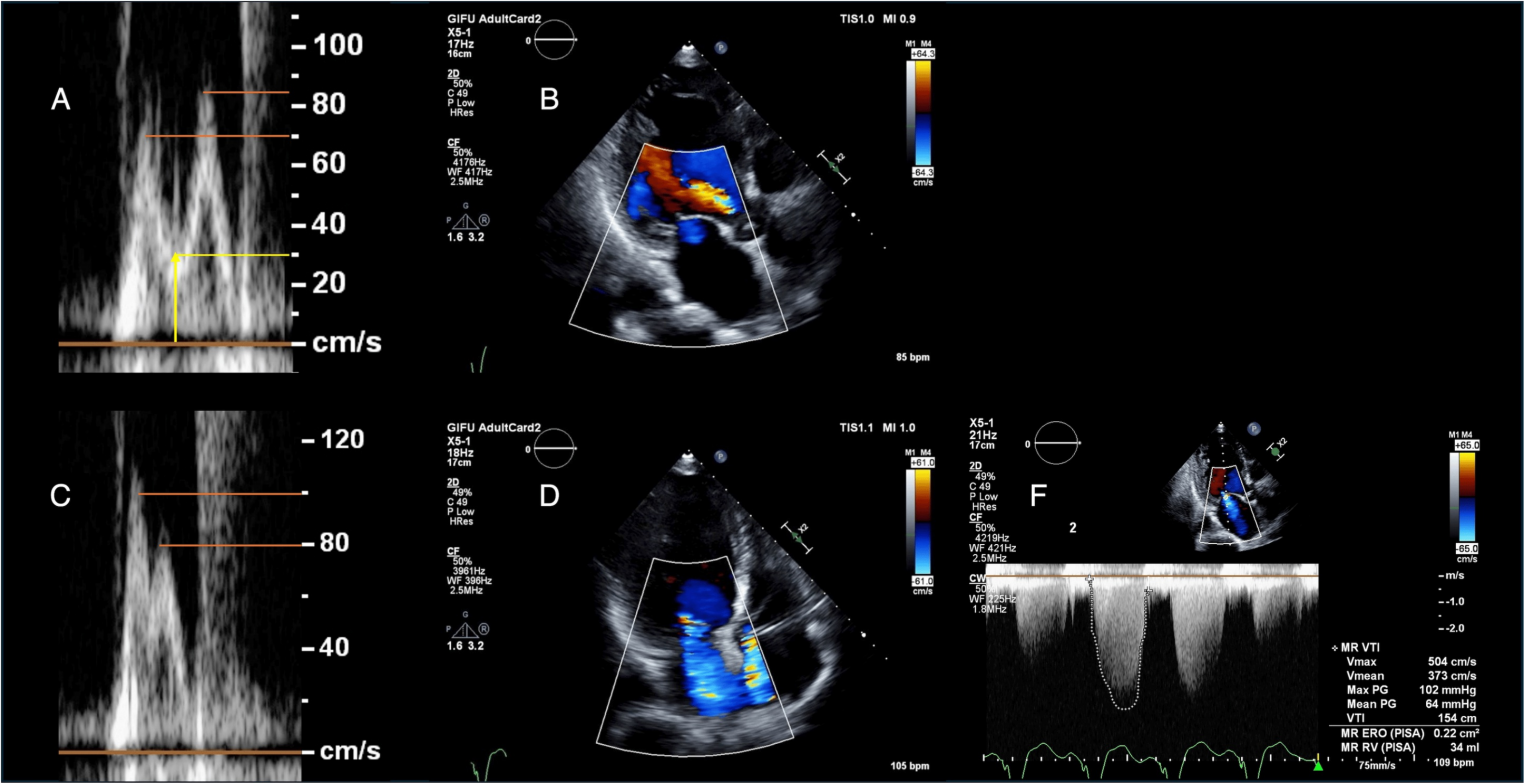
Preoperative exercise echocardiography A: E and A wave velocities at rest; E wave: 70 cm/s; A wave: 85 cm/s, initial E/A ratio: 0.82, adjusted E/A ratio: 1.27, accounting for A wave onset at 30 cm/s (yellow line); B: trivial mitral regurgitation at rest; C: E and A wave velocities at 12.5 watts; D: severe mitral regurgitation at 12.5 watts; E: quantification of mitral regurgitation at 12.5 watts.
